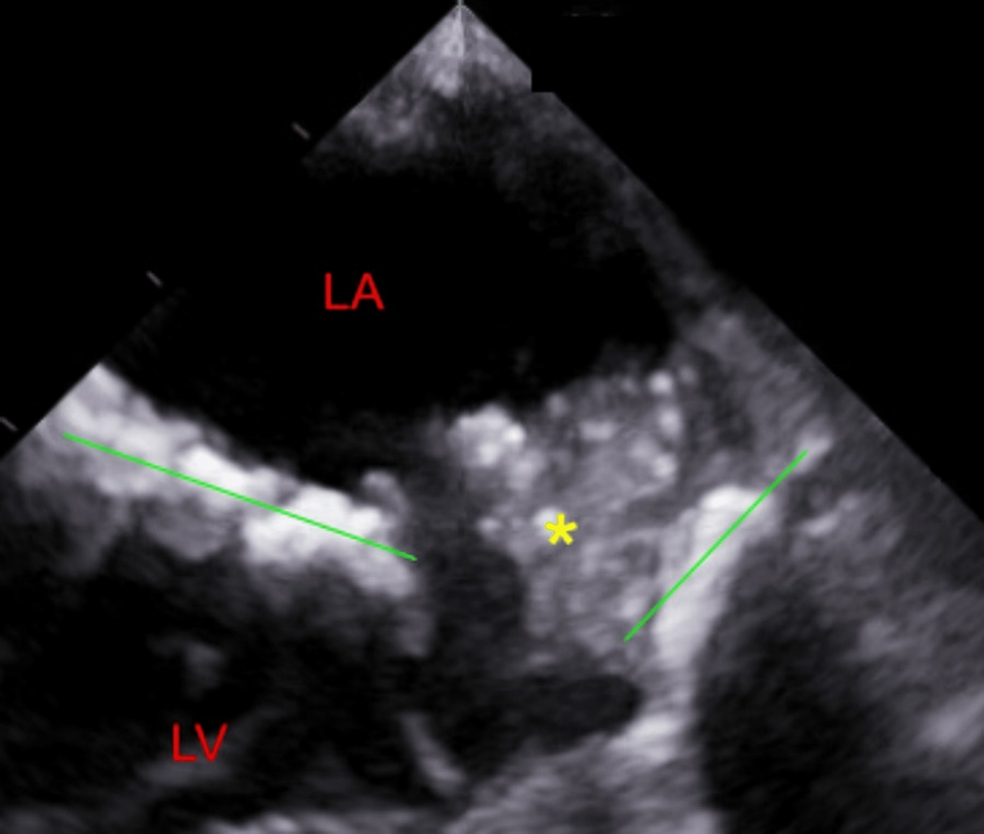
Image from transesophageal echocardiogram showing blockage of the mitral valve orifice (green lines) by a large vegetation (yellow asterisk) during atrial contraction.
